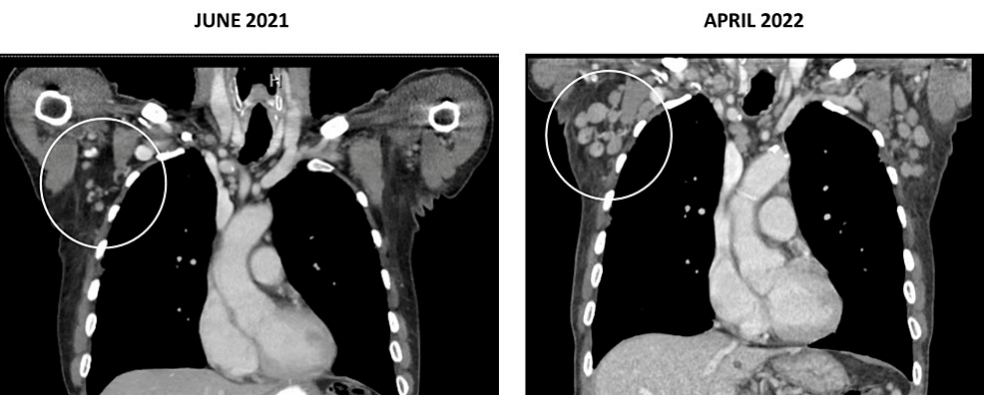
Chest CT scan showing the change in axillary lymphadenopathy from June 2021 to April 2022
